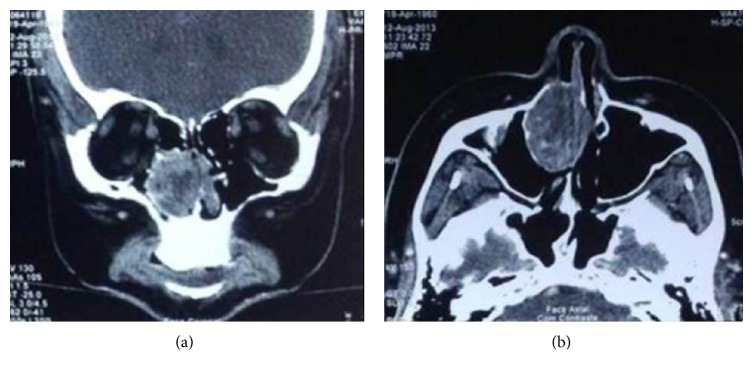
Computed tomography. Coronal (a) and axial (b) planes demonstrate heterogeneous contrast enhancement by the tumor.
